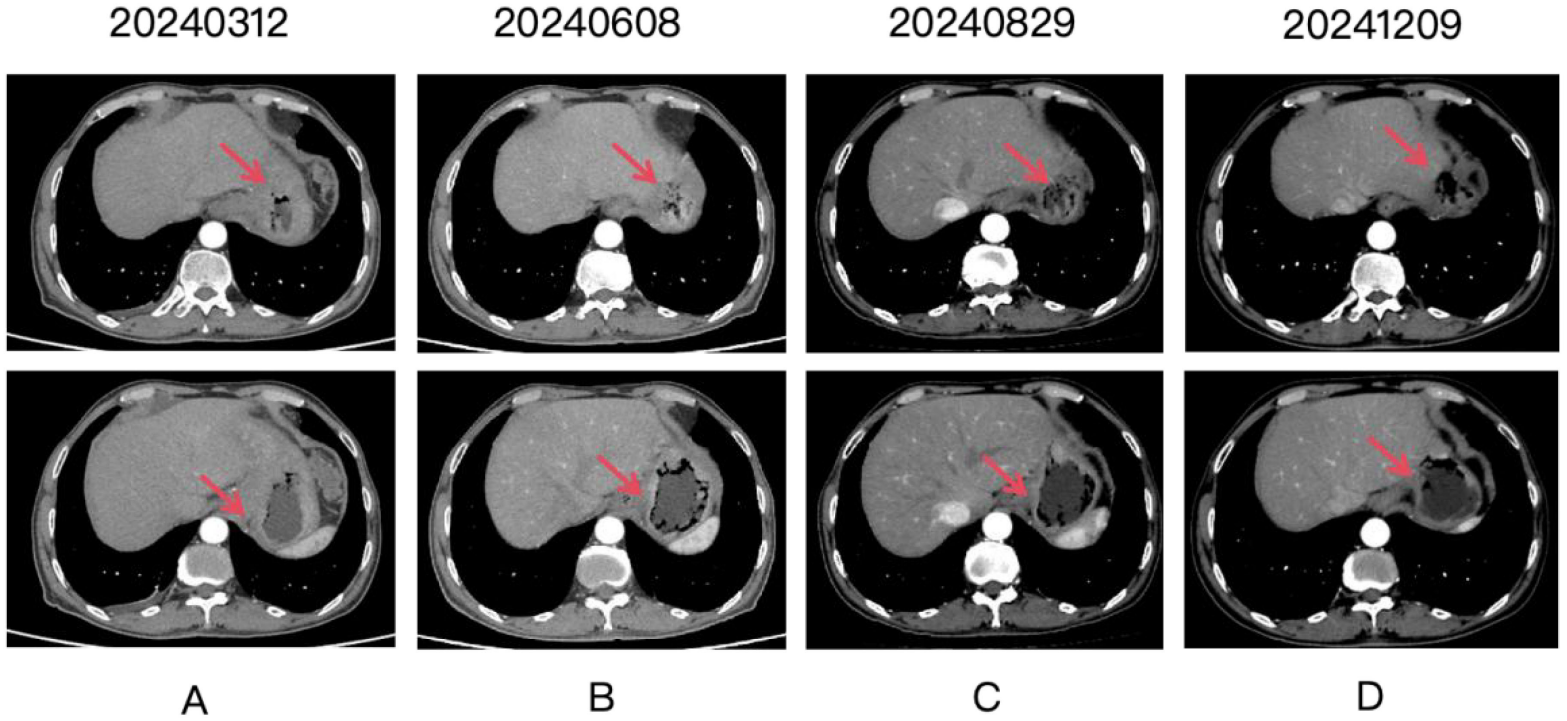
Four panels labeled (A-D) show CT scan images over time, with dates 20240312, 20240608, 20240829, and 20241209. Each panel contains two images. Red arrows point to the gastric lesion, which shows a partial response after treatment.
